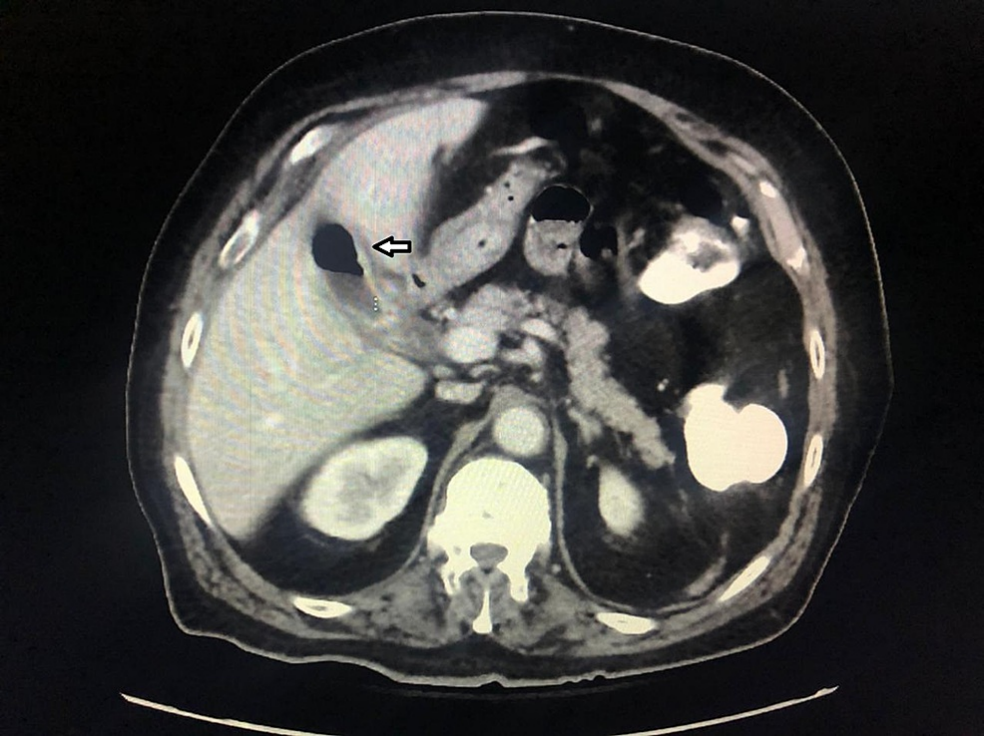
Abdomen CT with intravenous and oral contrast showing pneumobilia
